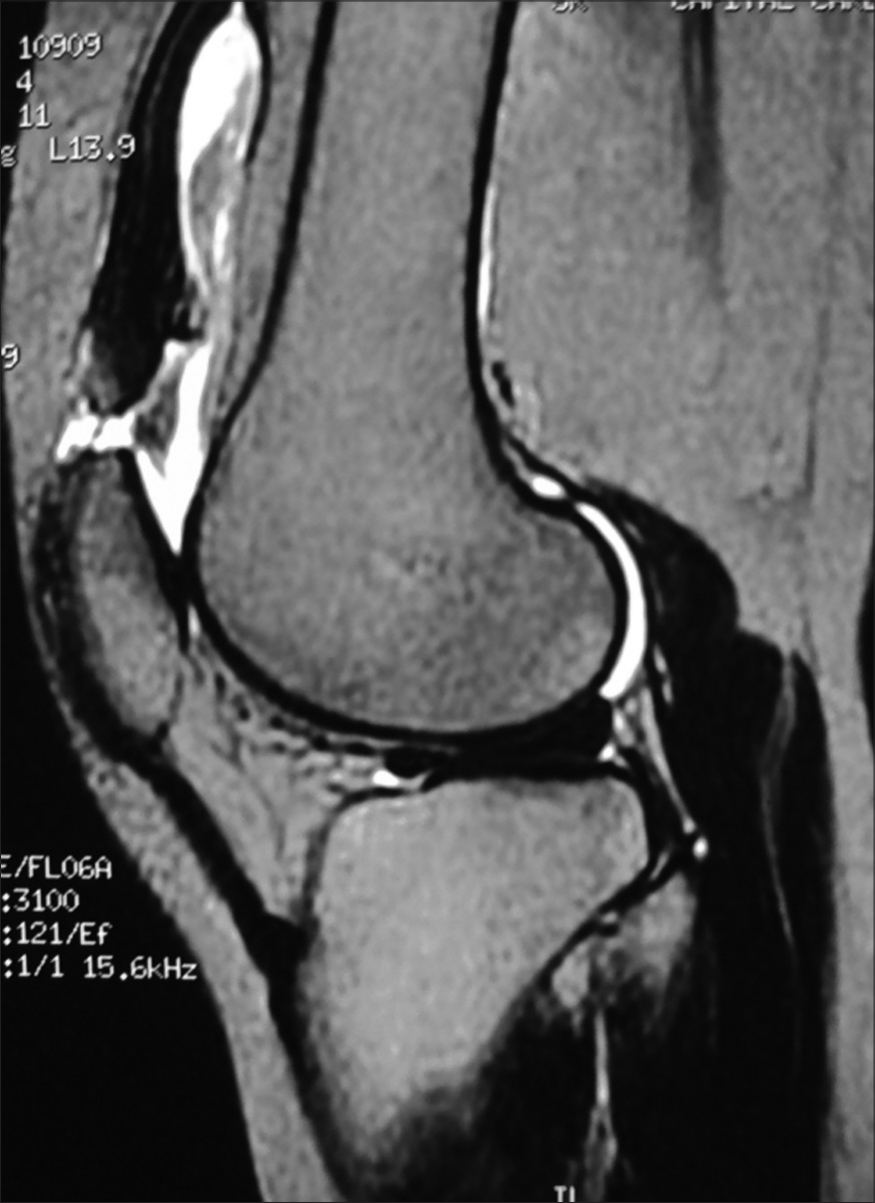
T2-weighted sagittal MR image of left knee joint shows discontinuity between quadriceps tendon and upper pole of patella filled with hyperintense signal intensity
